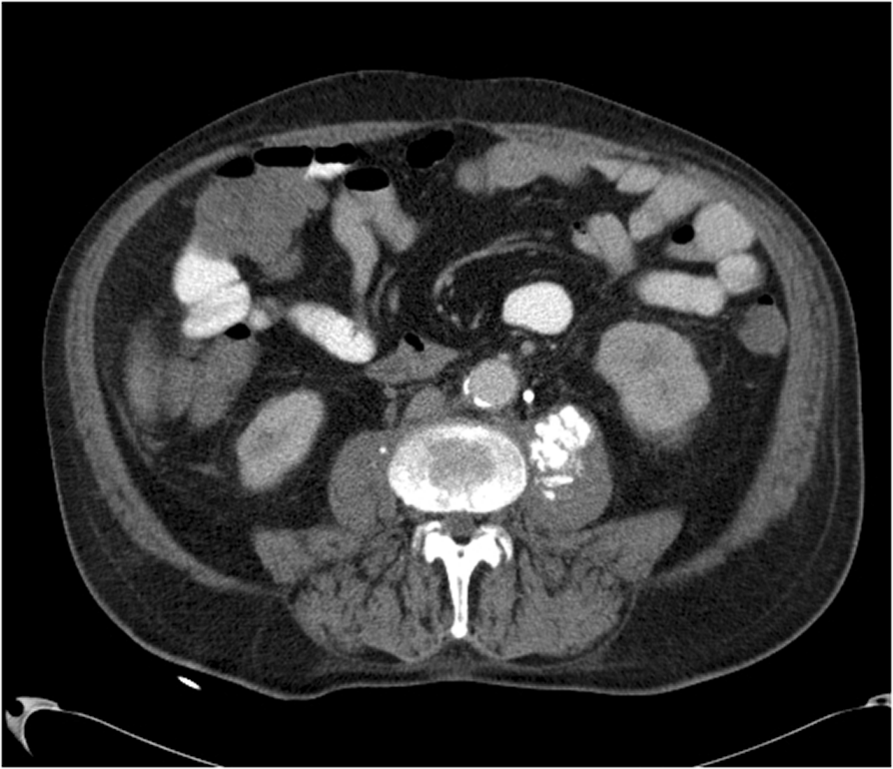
MM type IV in a patient with urinary bladder carcinoma. CT image demonstrates multiple calcifications in the left iliopsoas muscle (arrow).
